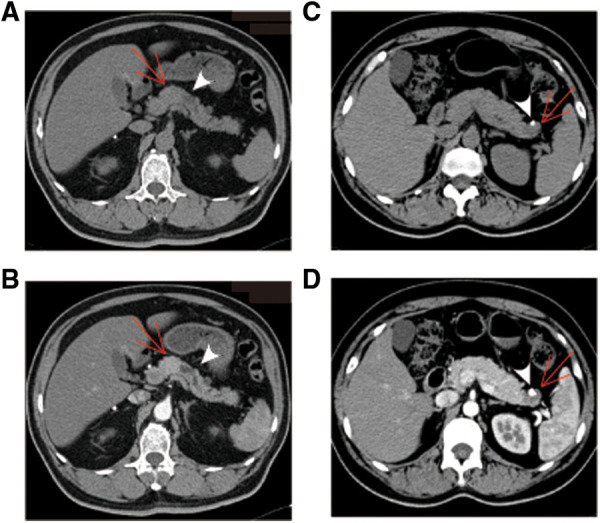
Typical CT images of pNET and SPT. (A,B) pNET in a 30-year-old man with no physical discomfort. Axial unenhanced CT image demonstrated a regular and well-defined solid mass in the neck of the pancreas [(A), arrow]. The mass was displayed as hyperattenuation and homogenous density in arterial phase of contrast-enhanced CT images (B). Apparent dilation of the distal pancreatic duct is observed (arrowhead). (C,D) SPT identified during physical examination in a 45-year-old woman. A cystic mass with low density and a well-defined margin was presented in the tail of the pancreas [(C), arrow]. The cystic portion was poorly enhanced in the arterial phase of contrast-enhanced CT images (D). Peripheral calcification was observed, with a size of 0.6 × 0.9 cm (arrowhead). pNET, pancreatic neuroendocrine tumor; CT, computed tomography; SPT, solid pseudopapillary tumor.
